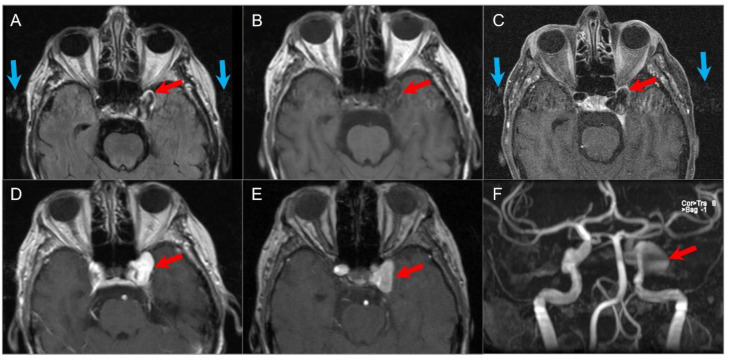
Giant left cavernous ICA aneurysm. (A) Axial FLAIR, (B) T1W, and (C) black-blood T1W vessel wall imaging show a giant left cavernous ICA aneurysm (red arrows) with pulsation artifact along the phase-encoding direction (blue arrows) and internal heterogeneous signal on FLAIR. (D) Axial postcontrast T1W image reveals homogenous enhancement of the aneurysm. (E) TOF MR angiography source and (F) MIP images show bright flow-related signal in the aneurysm.
